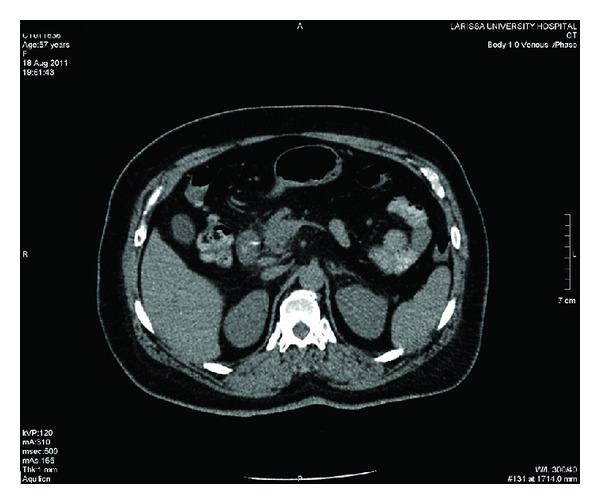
Contrast-enhanced CT image showing the foreign body in the duodenum as well as the presence of air outside the lumen.
